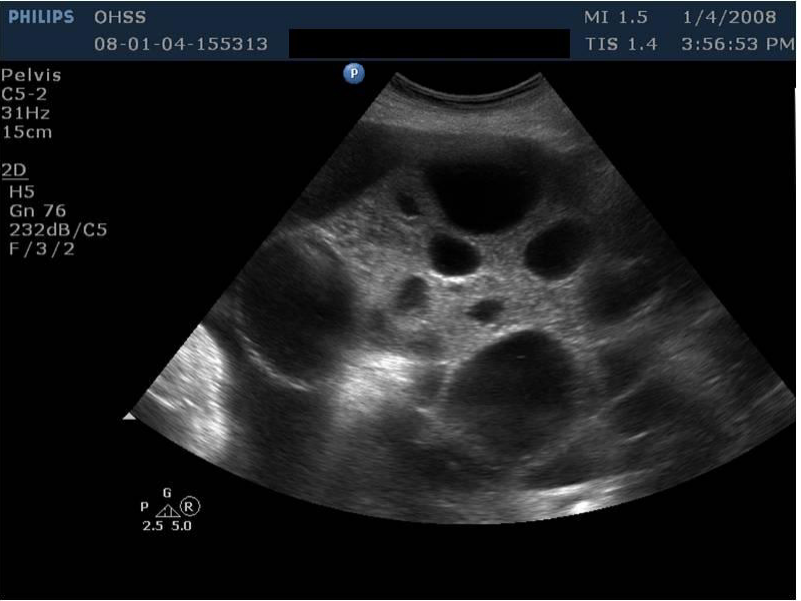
Ultrasonographic examination revealed bilaterally enlarged multicystic ovaries.
